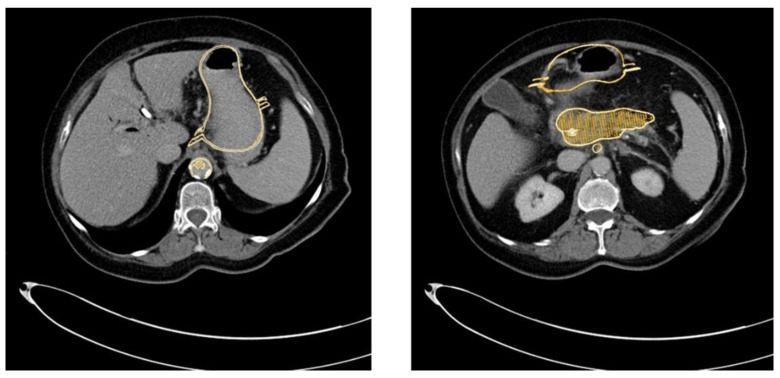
Overlay of the CT scan and the 3D-printed model (golden) and the original patient’s CT scan, demonstrating the high congruency between the replica and the original data.
